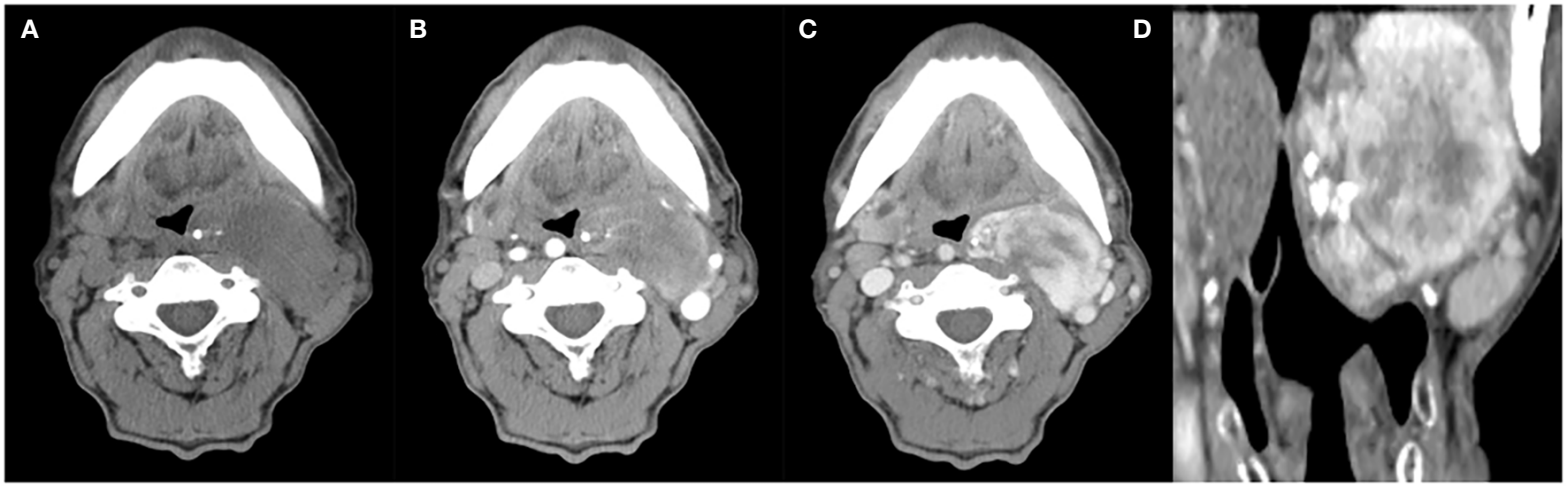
A 63-year-old man with SFT. (A) Precontrast CT scan shows an oval mass with calcification in the left parapharyngeal space. (B) Several enlarged intratumoral vessels are seen in the arterial phase on post-contrast CT image. (C, D) Heterogeneous marked enhancement is detected in the venous phase.
